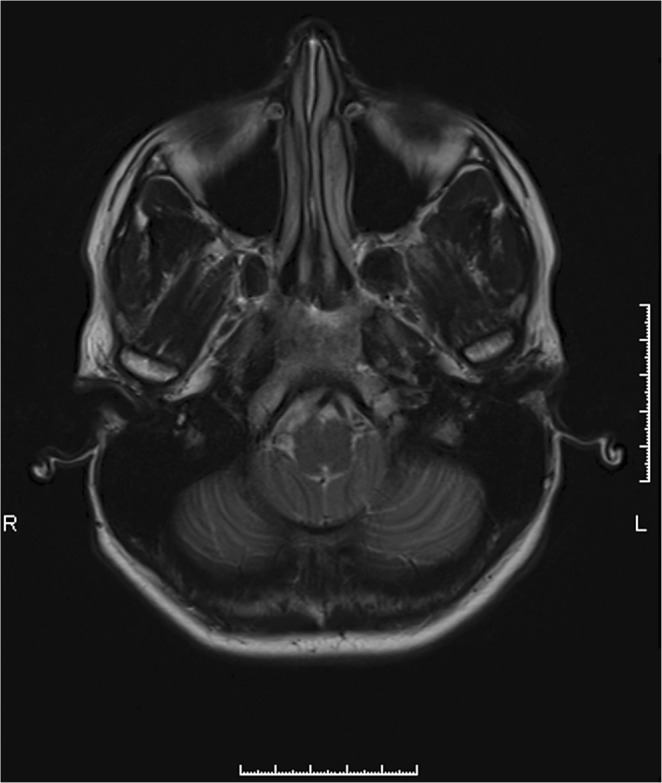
Axial view of the horizontal plane focusing optimum depiction of mandibular condyles. This MRI slice does not show the most superior condylar points used for measurements.
